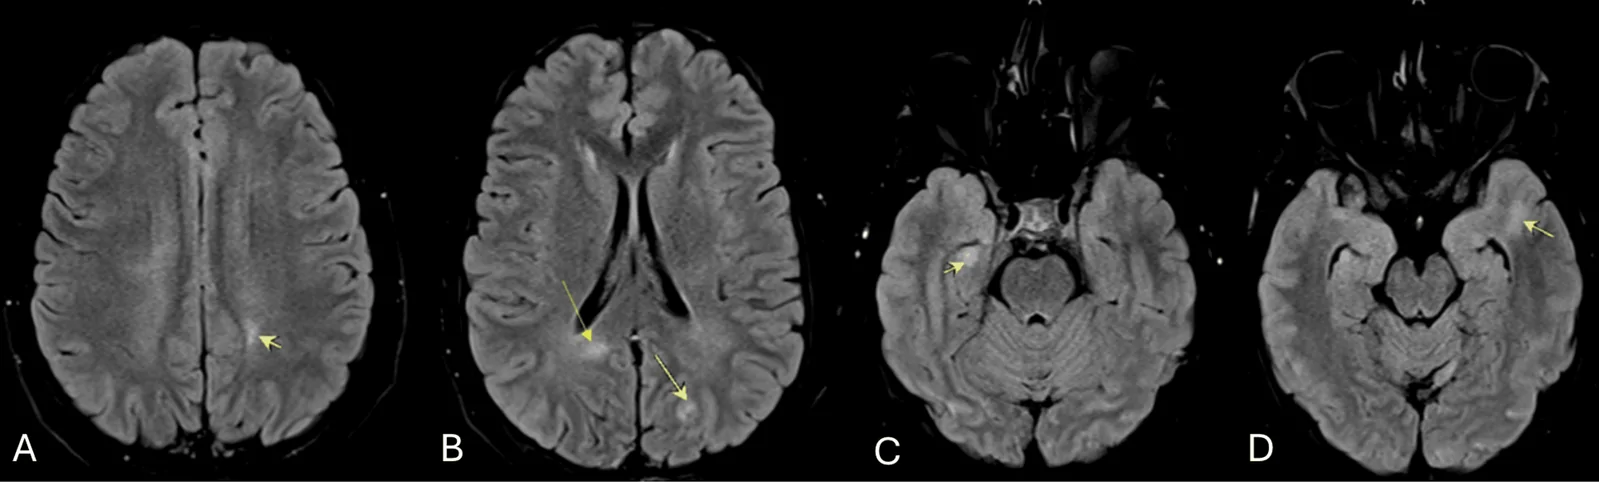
Brain MRI demonstrating multifocal demyelinating lesions FLAIR MR images at multiple brain levels demonstrate multifocal white matter lesions consistent with a central nervous system demyelinating process (A-D). The yellow arrows indicate the multifocal demyelinating lesions. MRI: magnetic resonance imaging; FLAIR: fluid-attenuated inversion recovery
